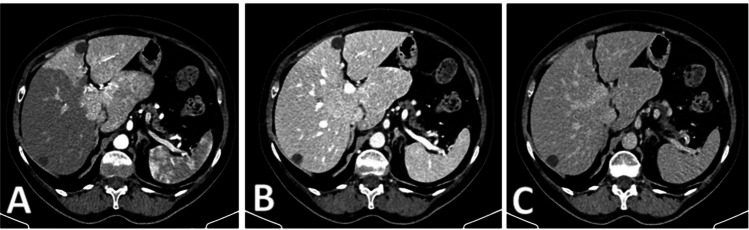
Abdominal Computed tomography with contrast medium: there is an enlarged liver with a pathological mass involving the entire caudate lobe and parts of the adjacent right segments, measuring up to 10 cm in maximum diameter. On contrast medium, it was characterized by inhomogeneous contrast enhancement (A), thus appearing slightly hypodense in the venous and late phases (B and C), with multiple markedly hypodense areas within it. The mass exerted significant compression on the hepatic veins at their confluence with the inferior vena cava, leading to hypodense appearance in the right hepatic lobe during the arterial phase due to altered blood supply. Multiple small hypodense lesions of up to 7 mm in size were observed in the remaining liver parenchyma
